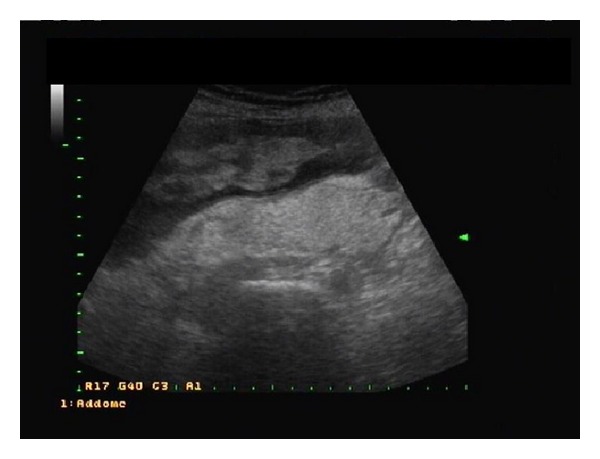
Abdominal ultrasonography showing a solid, heterogeneous retroperitoneal mass with multiple foci of variable size, occupying most of abdominal and pelvic cavity.
